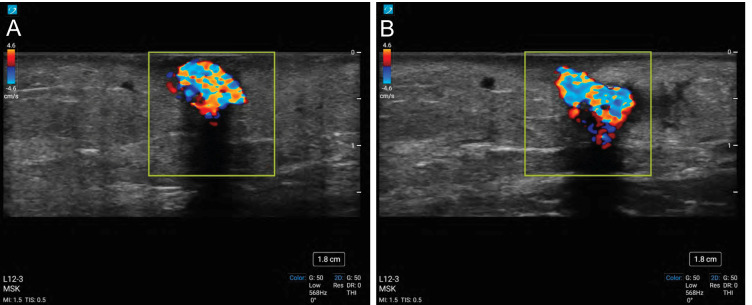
Twinkle artifact viewed withcolour Doppler scanning of the myiasis in short axis (A) and in long axis (B).
